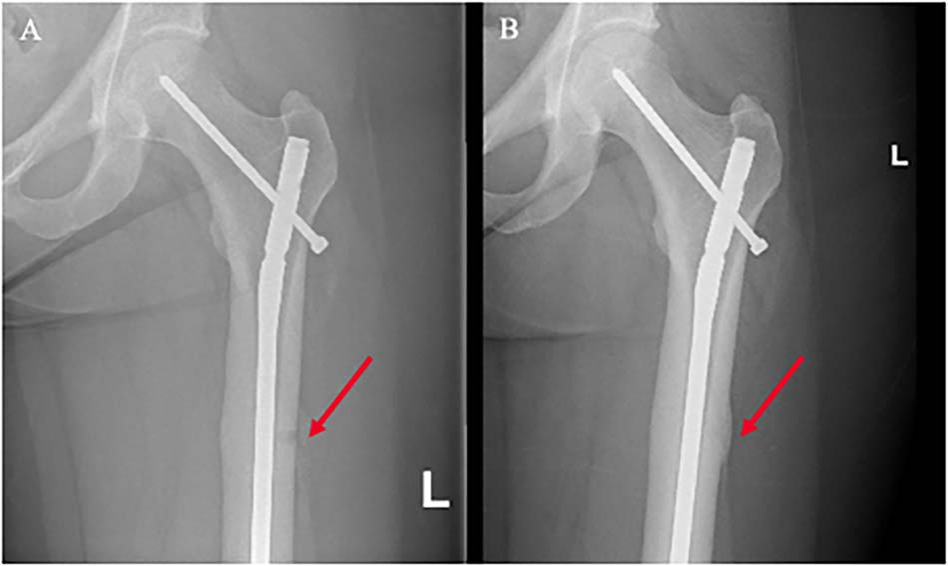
Serial X-rays of the left proximal femur 7 mo apart demonstrate (A) an insufficiency fracture and (B) evidence of fracture resolution and callus formation on burosumab.
